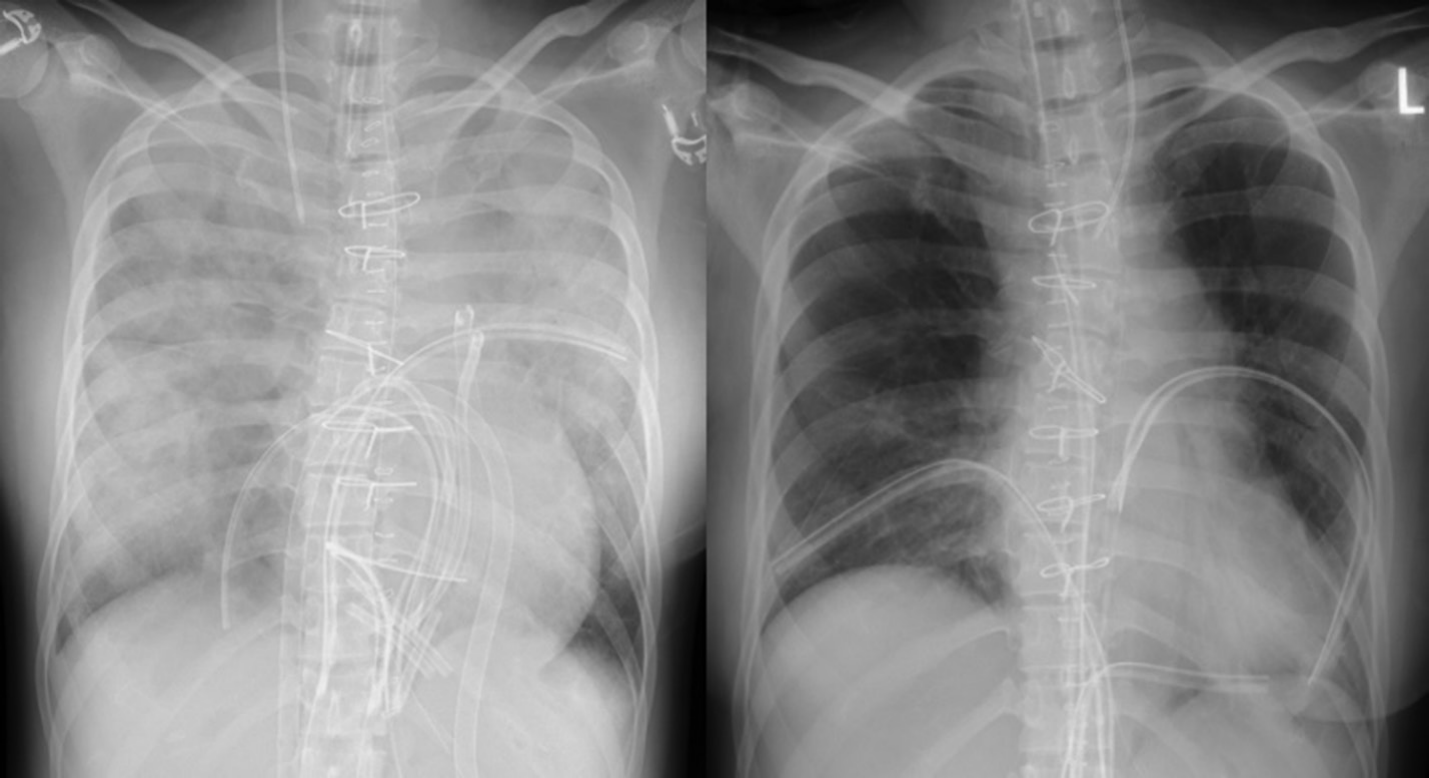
Chest radiograph at the introduction and weaning of ECMO. Lung field permeability improved. (Left: introduction, right: weaning).
